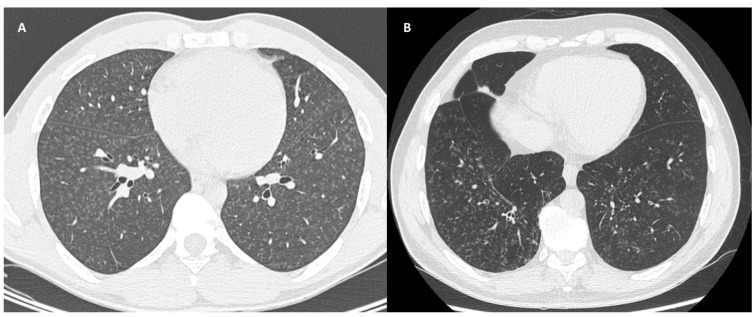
Chest HRCT, axial planes, patient affected by talc granulomatosis (A) and patient affected by aspiration pneumonia (B). In both (A,B), CT shows numerous millimetric micronodules in a centrilobular pattern. The differential diagnosis between aspiration pneumonia and talc granulomatosis, when occlusive bronchus involvement and pulmonary atelectasis are not present, can be very challenging and impossible without a detailed clinical history, bronchoscopy, and BAL.
